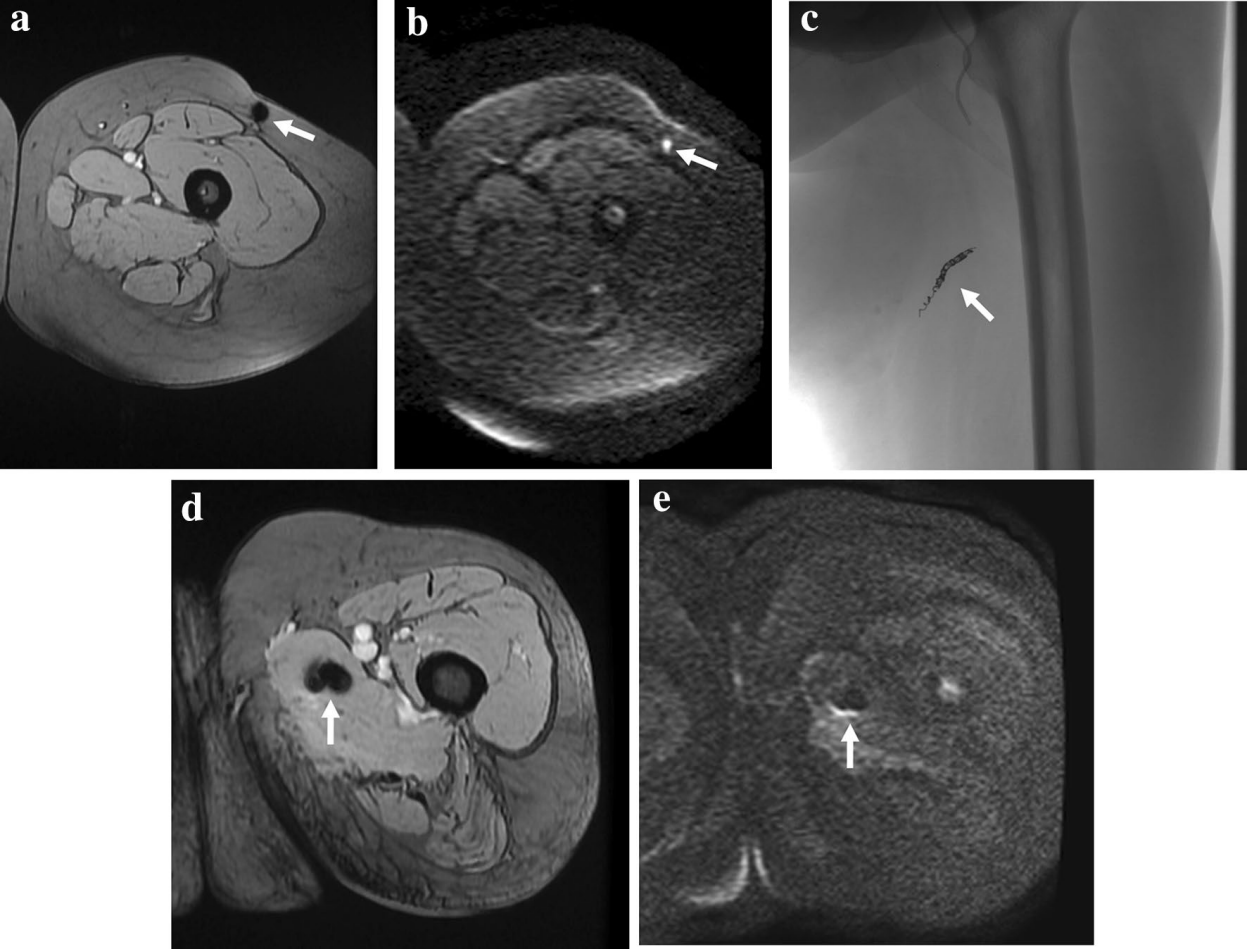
Two pictorial examples of ferromagnetic artifacts due to metallic objects of different sizes. Forty-year-old woman, MR follow-up after surgical excision of synovial sarcoma in the left thigh. (a, b); a Axial T2*w GRE shows a small artifact (*arrow*) due to metallic particles in the scar (blooming artifact). b Axial DWI shows a focal pseudonodular hyperintensity at the level of the metallic particle (*arrow*) without the typical signal void, possibly leading to diagnostic misinterpretation. Sixty-three-year-old woman, MR follow-up after surgical excision of pleomorphic sarcoma (c, d, e). c The fluoroscopic anteroposterior projection shows the presence of metallic spirals (*arrow*) due to pre-operative embolization. Axial d T2*w GRE and e DWI reveal the typical artifacts due to metallic spirals (*arrow*), emphasized by the T2* weighting
